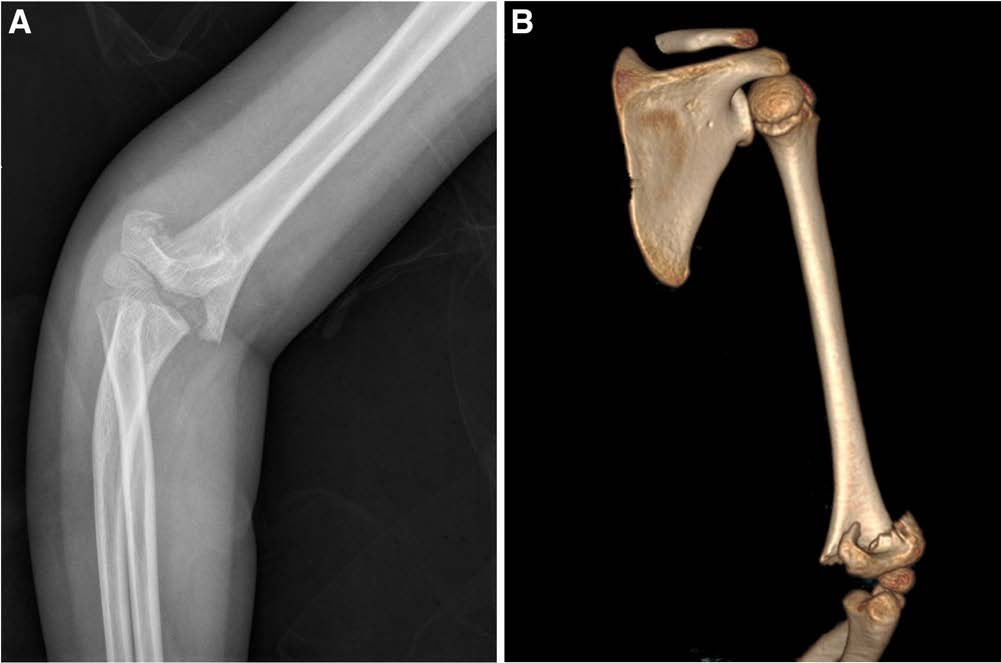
Imaging findings of supracondylar fractures of the humerus. A: X-ray; B: CT. CT = computed tomography.
